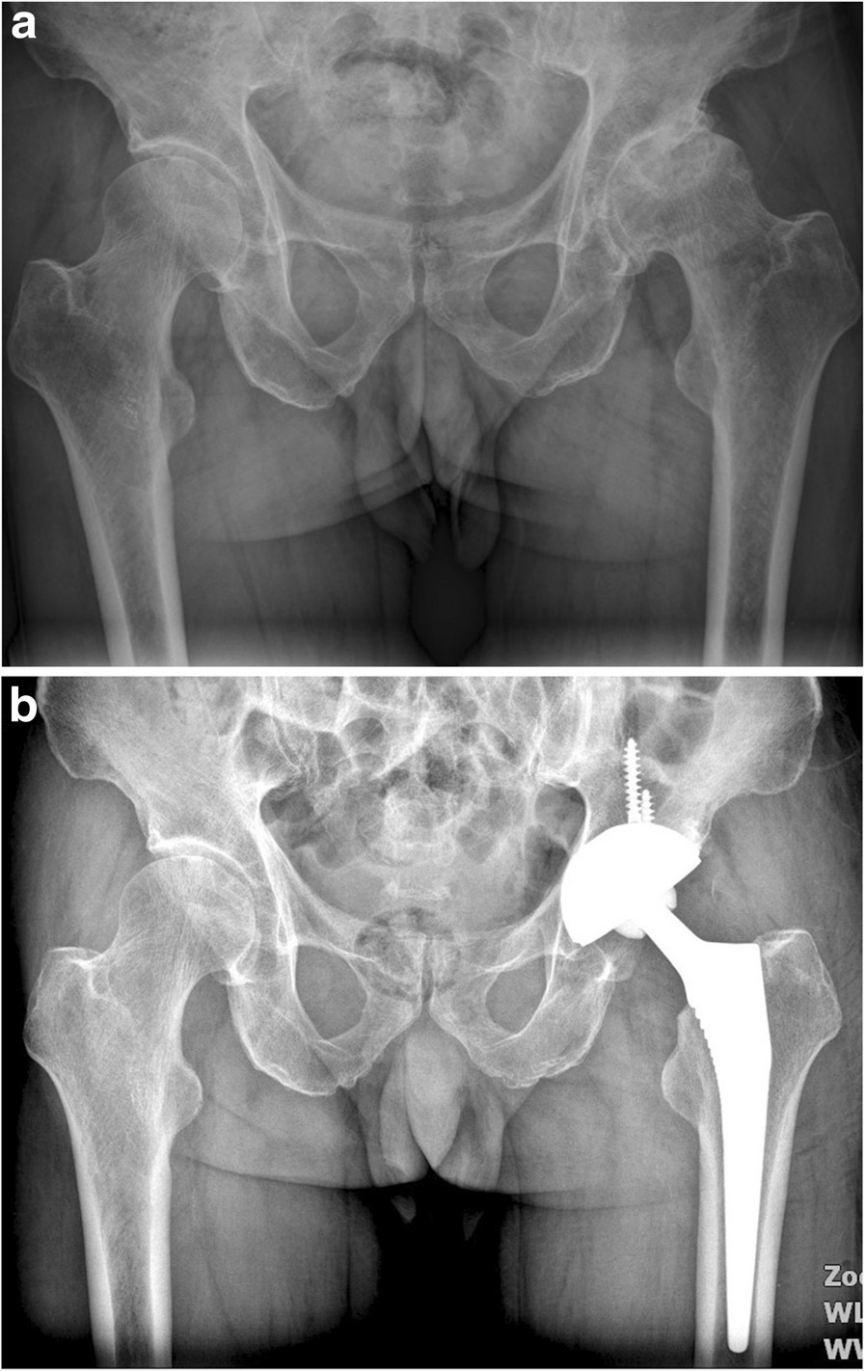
a A preoperative radiograph of the hip of a 76-year-old man who had left hip osteoarthritis. b A radiograph at 40 months after implantation of conventional cementless femoral stem showed solid fixation in a satisfactory position
